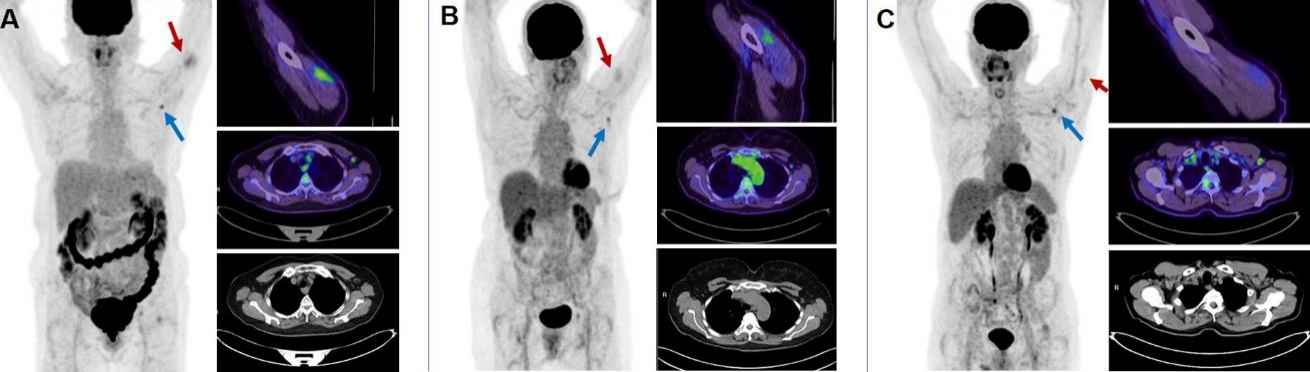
Vaccine-related ^18^F-FDG PET/CT features on day one (A), seven (B) and twenty-eight (C) after AstraZeneca vaccination. As seen on the image, the deltoid uptake reduces over time, however, the ipsilateral axillary nodal uptake remains stable over the first four weeks post vaccination. (A)A 60-year-old female with adenocarcinoma of the cervix 6-month post completion of brachytherapy presents for restaging. There was a minor increase in uptake through the musculature of the left upper arm laterally (SUV_max_=2.9) (red arrow) as well as in a mildly enlarged (11 mm) left axillary lymph node (SUV_max_=3.7) (blue arrow), consistent with recent COVID vaccination. She had received first dose of AstraZeneca one day prior to the study. (B)A 70-year-old female with right lower lobe ground glass changes for assessment. Two hypermetabolic left sided axillary lymph nodes (SUV_max_=3.4) (blue arrow) and increased posterior left deltoid uptake (SUV_max_=2.0) (red arrow) are related to AstraZeneca vaccination 7 days prior to imaging. (C)A 68-year-old male with a background history of follicular lymphoma, who recently has presented with multiple pulmonary nodules, for further evaluation. Few non-enlarged tracer avid lymph nodes in the left axilla (blue arrow) were noted (SUV_max_ up to 4.3). Mild FDG activity in the left deltoid muscle (red arrow) corresponds to the injection site of recent COVID vaccine. The patient had AstraZeneca vaccination 28 days prior to imaging
